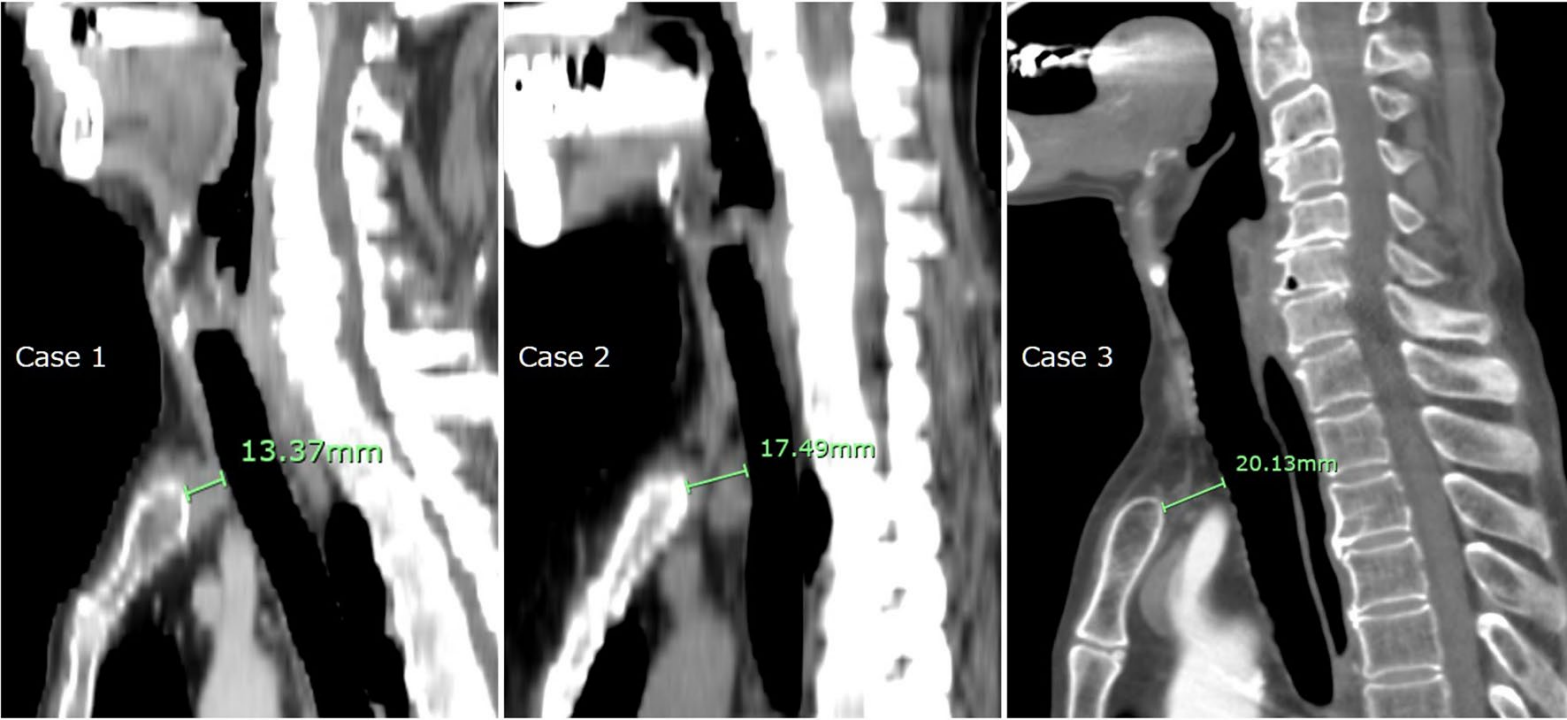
Measurement of the sterno-tracheal distance (STD) in each case. The STD was defined as the minimum distance from the sternum to the anterior wall of the trachea in the sagittal section of cervical chest on preoperative computed tomography scans. None of the cases had an STD < 13 mm
